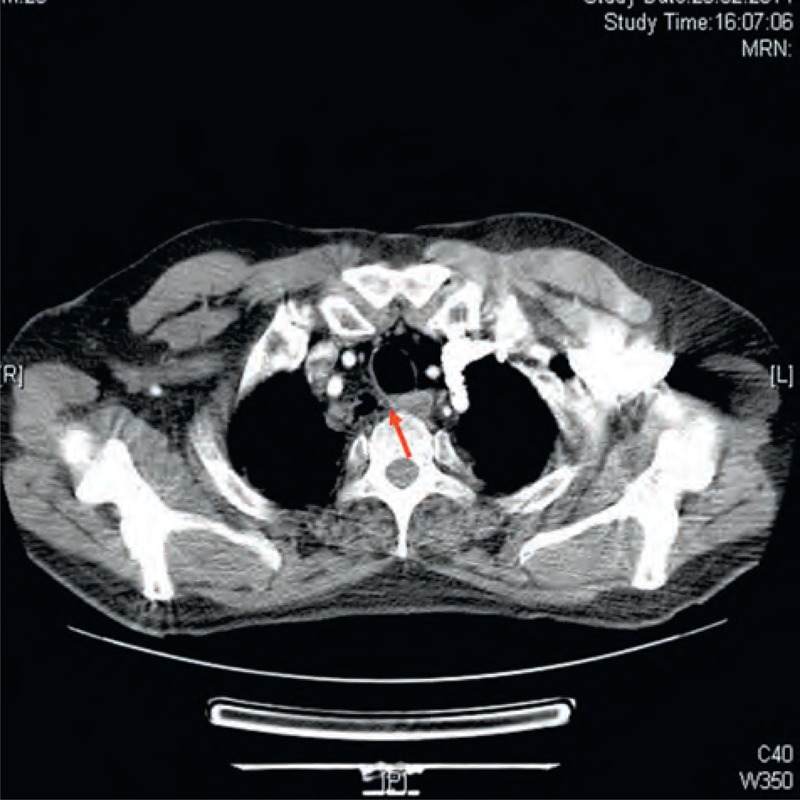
Thoracic computed tomography revealed regression of the lesion after closure of the temporalis muscle flap using argon plasma coagulation (arrow indicates that the connection between the cystic lesion and the trachea disappeared).
